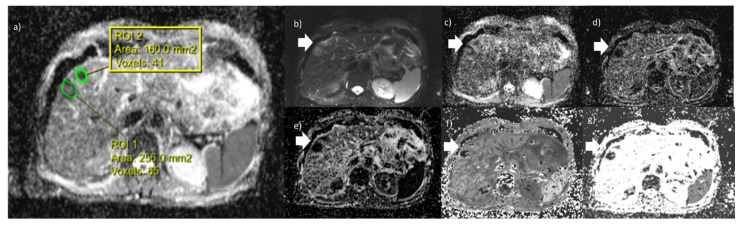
Representative case with two liver metastases and all DWI- and DKI-derived parameters maps: ADC map (a), S0 map (b), DT map (c), DP map (d), FP map (e), MK map (f), MD map (g).
